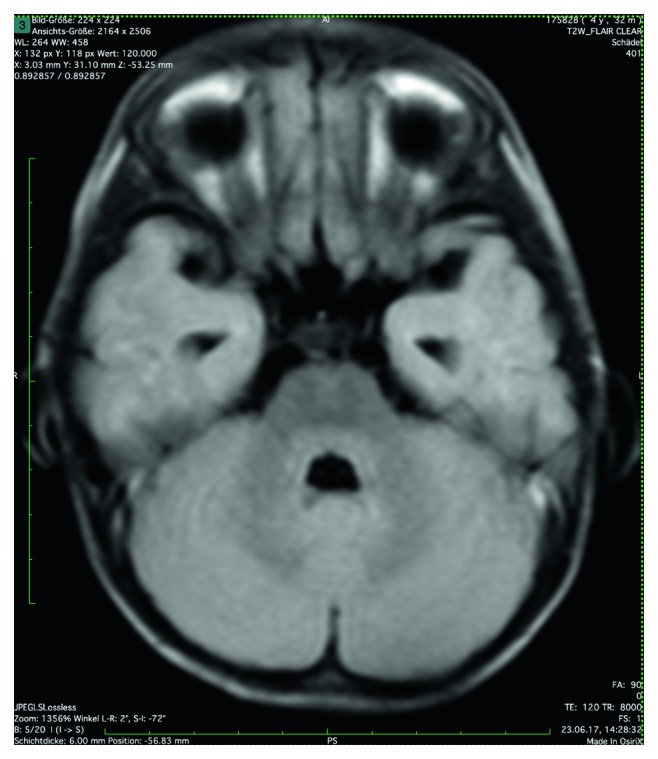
T2-FLAIR—unremarkable pons.
